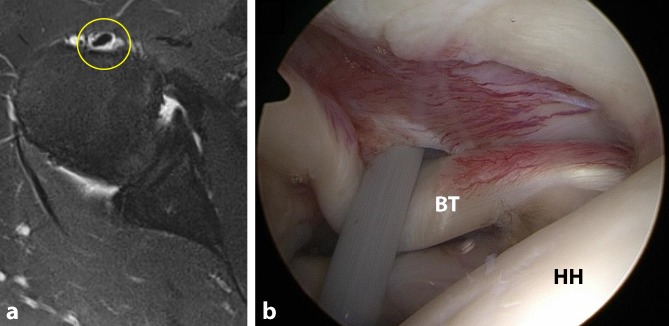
Images of a 46-year-old man with right-sided biceps tendonitis, diagnosed via history, physical examination, and a T2-weighted magnetic resonance imaging with a clear halo sign (*yellow circle*) around the long head of the biceps tendon indicating inflammation. The patient was treated conservatively with physical therapy and NSAIDs but continued to experience symptoms 6 months later. He thus underwent operative management as seen in b with the long head of the biceps tendon (*BT*) and biceps reflection pulley visualized through the standard posterior viewing portal. *HH* humeral head
